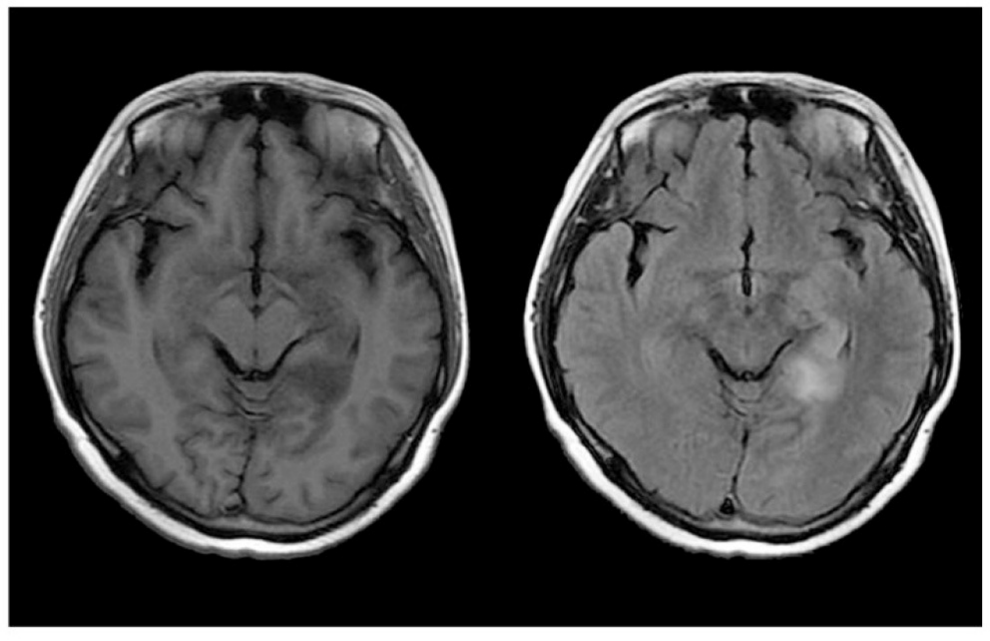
Neuroradiologic MRI (3.0 T) of Patient 2. The left temporal lobe and hippocampus showed hypointensity on T1WI and hyperintensity on FLAIR.
